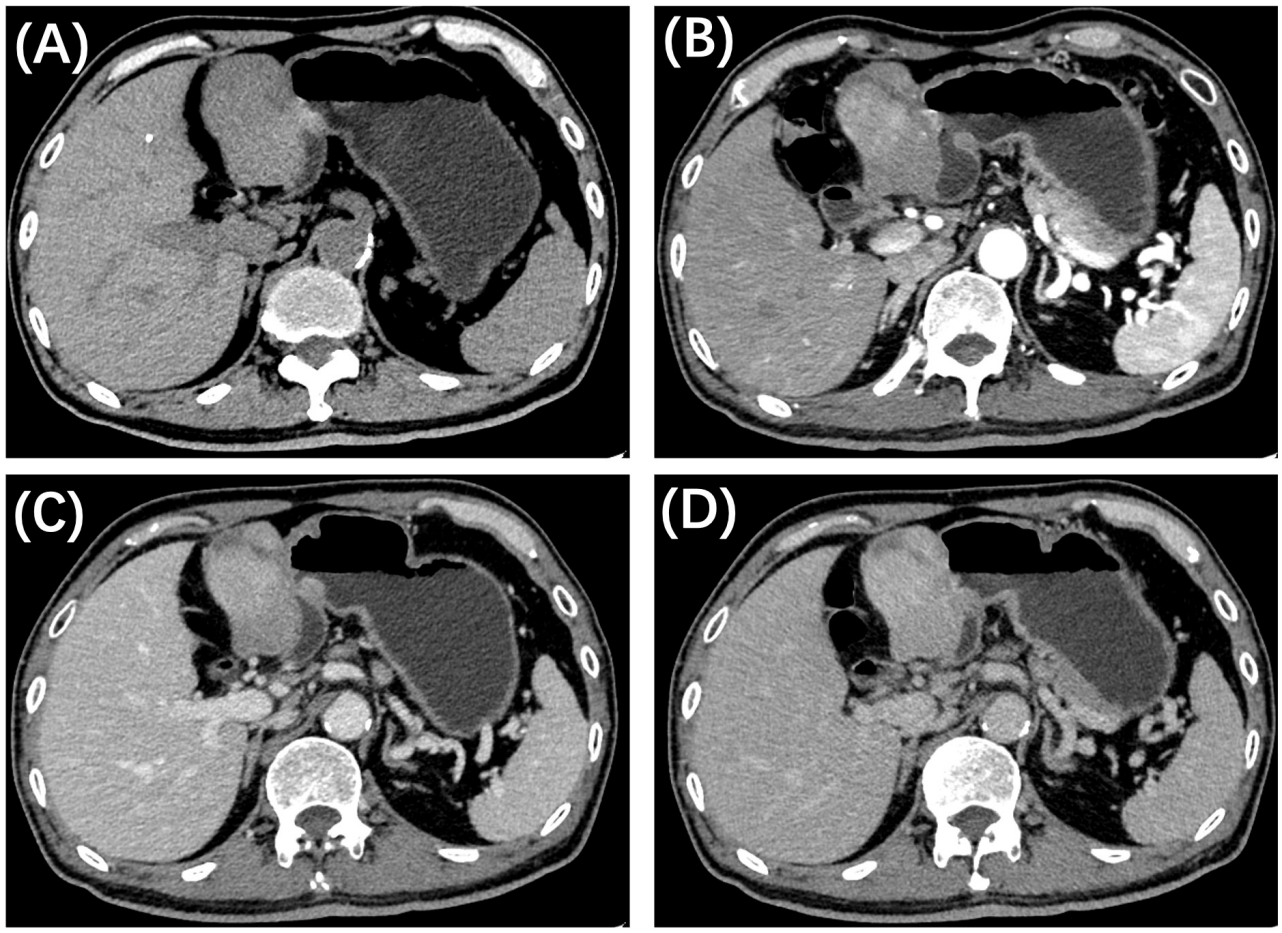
CT imaging of the present patient with primary hepatic leiomyosarcoma. (A) Axial precontrast CT reveals a well-defined, iso-dense mass in the left lobe of the liver, featuring a large exophytic component. Contrast-enhanced CT demonstrates uneven mild to moderate enhancement in the arterial phase (B), with gradual enhancement observed during the portal (C) and delayed (D) phases.
